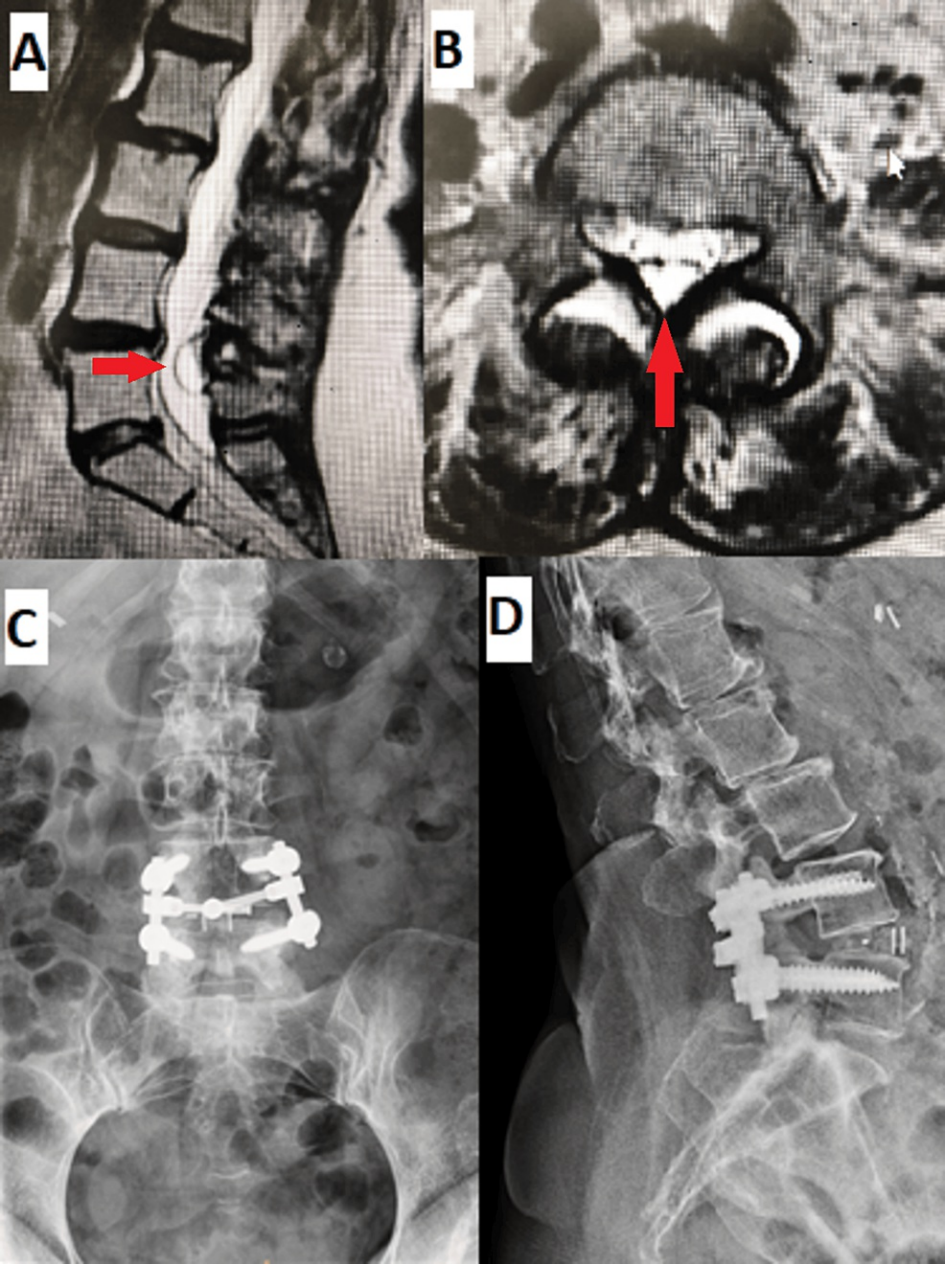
A 63-year-old female with a two-year history of low back pain associated with lower extremity radicular pain secondary to posterior epidural cyst causing neurogenic claudication treated with posterior decompression and fusion. (A) Sagittal MRI and (B) axial MRI showing the epidural cyst (red arrows). (C, D) Postoperative x-rays after posterior decompression and fusion.
